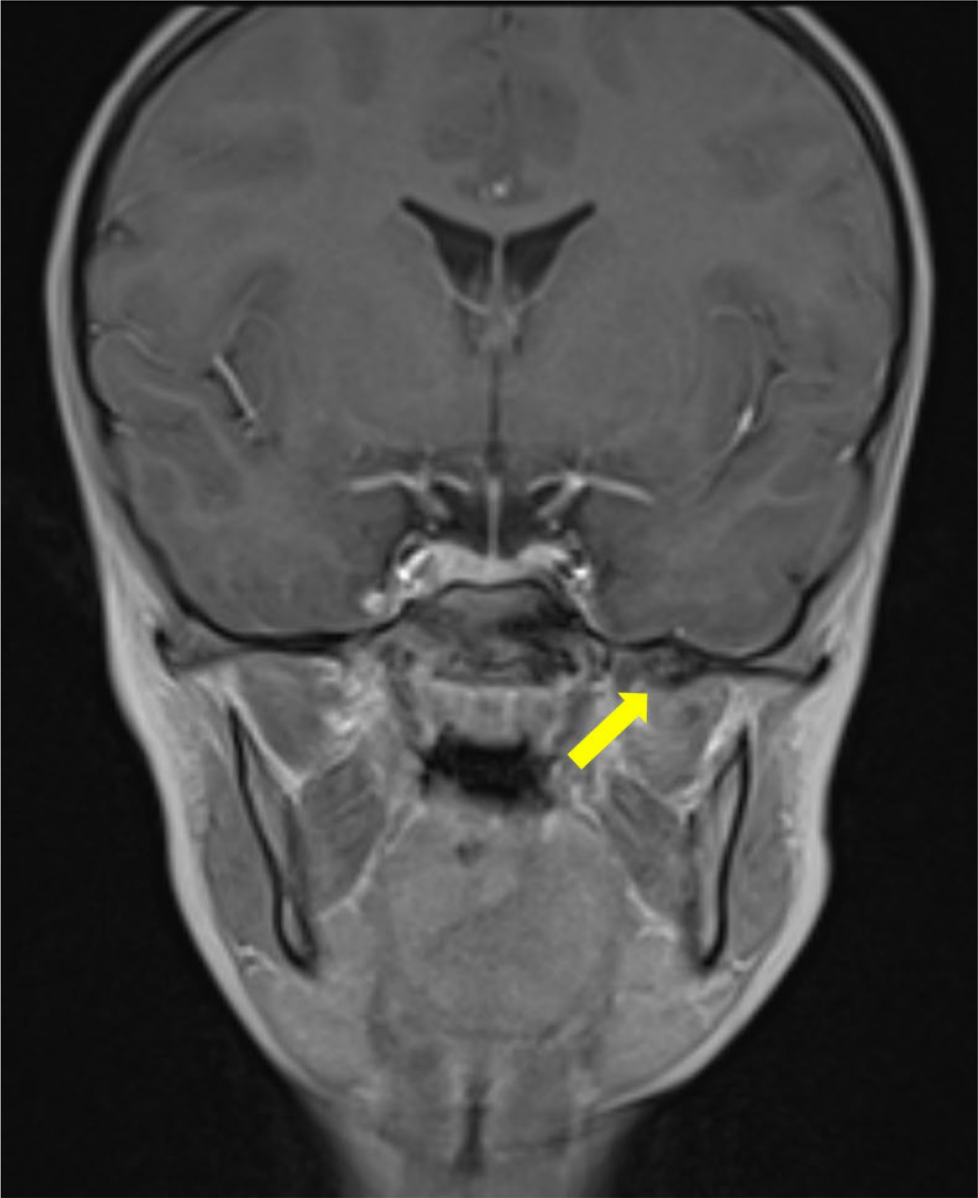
Contrast enhanced coronal T1 Dixon MRI scan showing the bony signal of the bony structure of the sphenoid spine (arrow) with no enhancement on the fat‑suppressed reconstructions (not shown).
